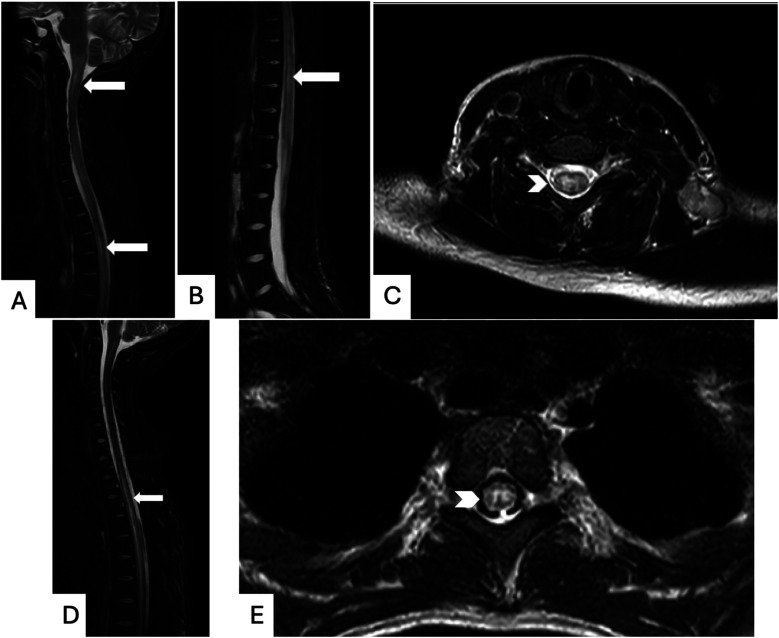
Magnetic resonance imaging (MRI) of the spine: patient 1: T2-weighted MRI images without contrast demonstrating longitudinally extensive T2 hyperintensities in the cervicomedullary junction through cervical and thoracic (A) and lumbar (B) spinal segments (long white arrows), as well as hyperintense signal on axial MRI cervical spine (C) affecting both gray and white matter (thick white arrowhead). Patient 2: T2-weighted Fluid-attenuated inversion recovery (FLAIR) sequence MRI images with Gadolinium contrast demonstrating extensive T2 hyperintensities in the thoracic spinal cord both on sagittal (D) and axial (E) views, demonstrated with long white arrows and thick white arrowhead, respectively.
